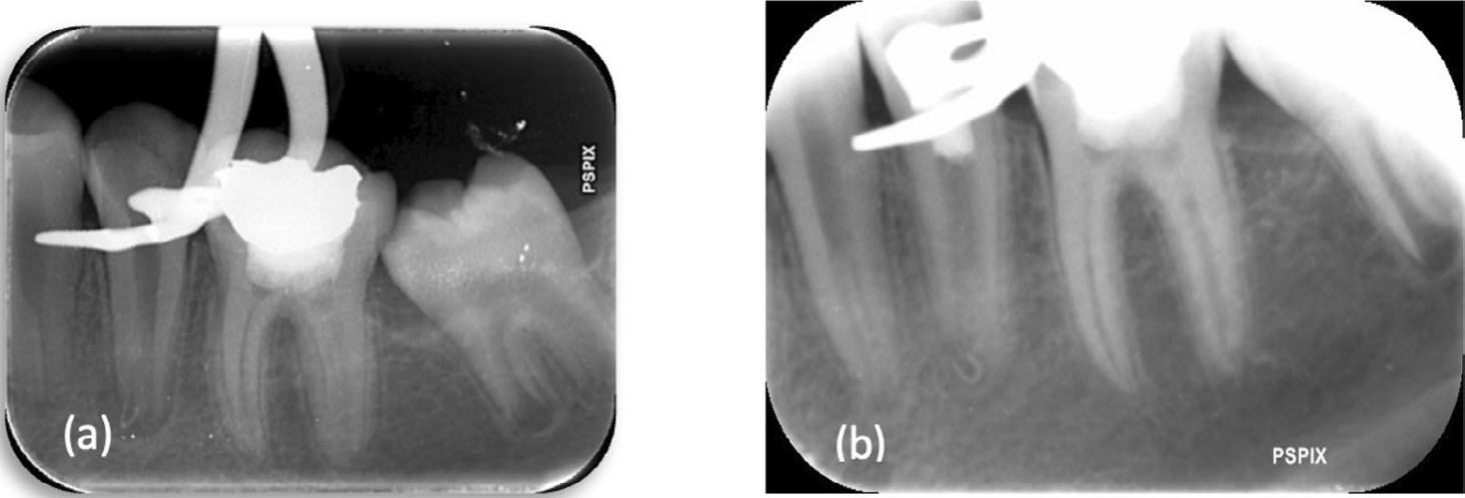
(a) After gutta‐percha removal; (b) Post‐operative radiography.
